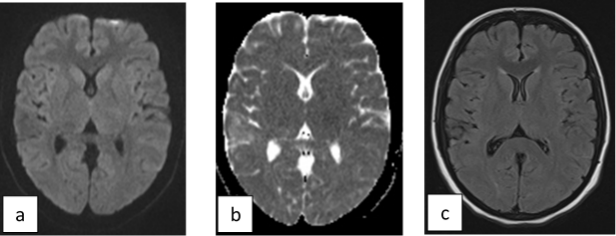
Normal MRI brain study of the patient on the first day of admission. Axial images at the level of thalami (a) axial DWI, (b) axial ADC map, (c) axial FLAIR. ADC, apparent diffusion coefficient; DWI, diffusion-weighted imaging; FLAIR, fluid-attenuated inversion-recovery.
